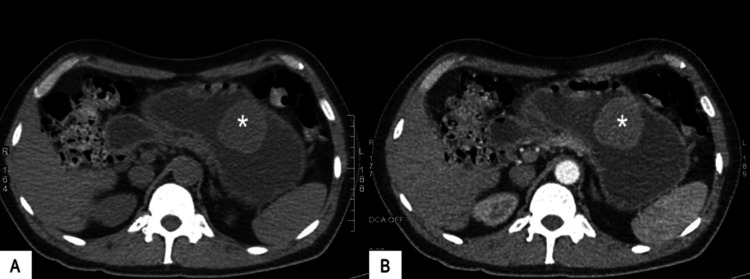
CT scan of the abdomen in the axial plane (A) CT scan of the abdomen without contrast revealing stomach-thickened walls and a large intragastric mass (asterisk) and (B) contrast-enhanced CT (arterial phase) showing the same lesion (asterisk). CT: Computed tomography.
